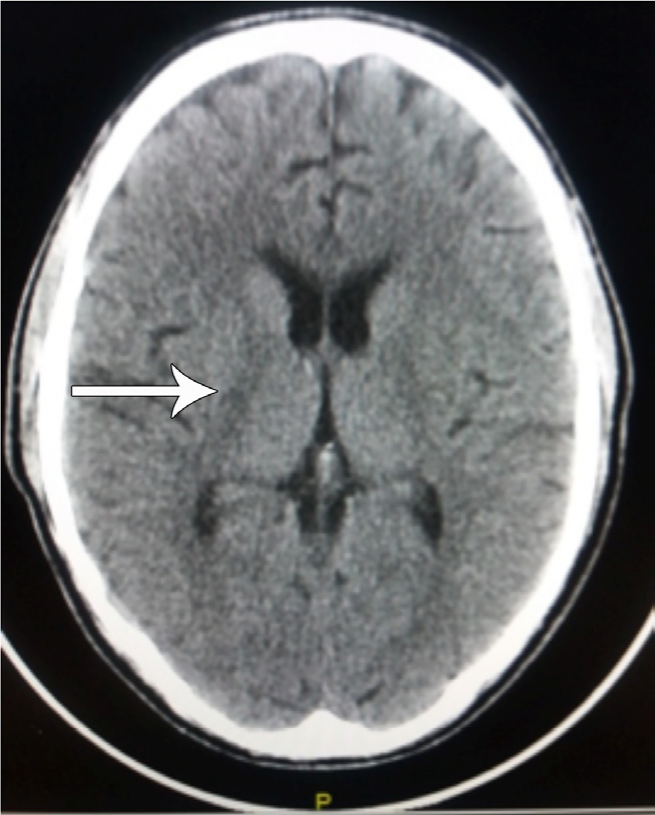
Computed tomography scan of brain at the time of developing PD symptoms (arrow).
